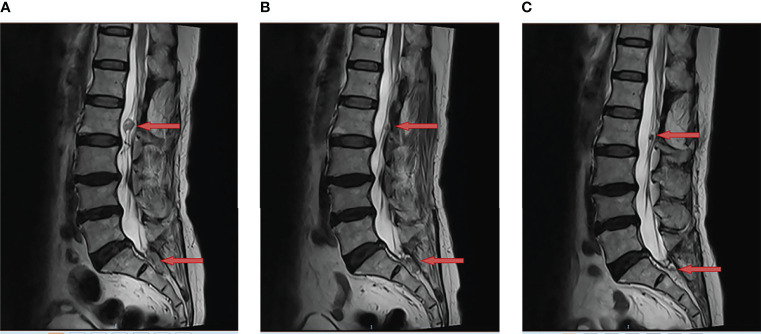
Anlotinib resulted in tumor regression of multiple recurrent lumbar and sacral cord hemangioblastoma. This is a sagittal magnetic resonance imaging (MRI) view of lumbar and sacral spine; (A) The two lesions at L2 and S1-S2 were observed to have increased in size (red arrow) in June 2021; (B) The MRI demonstrated tumor regression (red arrow) following one month of anlotinib in July 2021; (C) The MRI showed further regression in the enhancing tumor (red arrow) following three months of anlotinib in Sep 2021.
